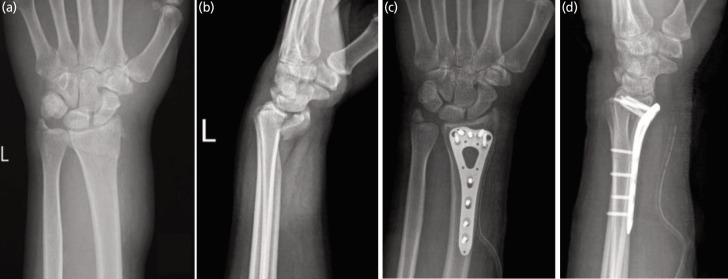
(a and b) Radiographs of 58-year-old female with an intra-articular radius fracture, (c and d) treated with volar locking plate fixation. Post-operative radiological measurements show radial height of 12.8mm, radial inclination of 19.6°, ulnar variance of – 1.9mm, and volar tilt of 5.6°.
